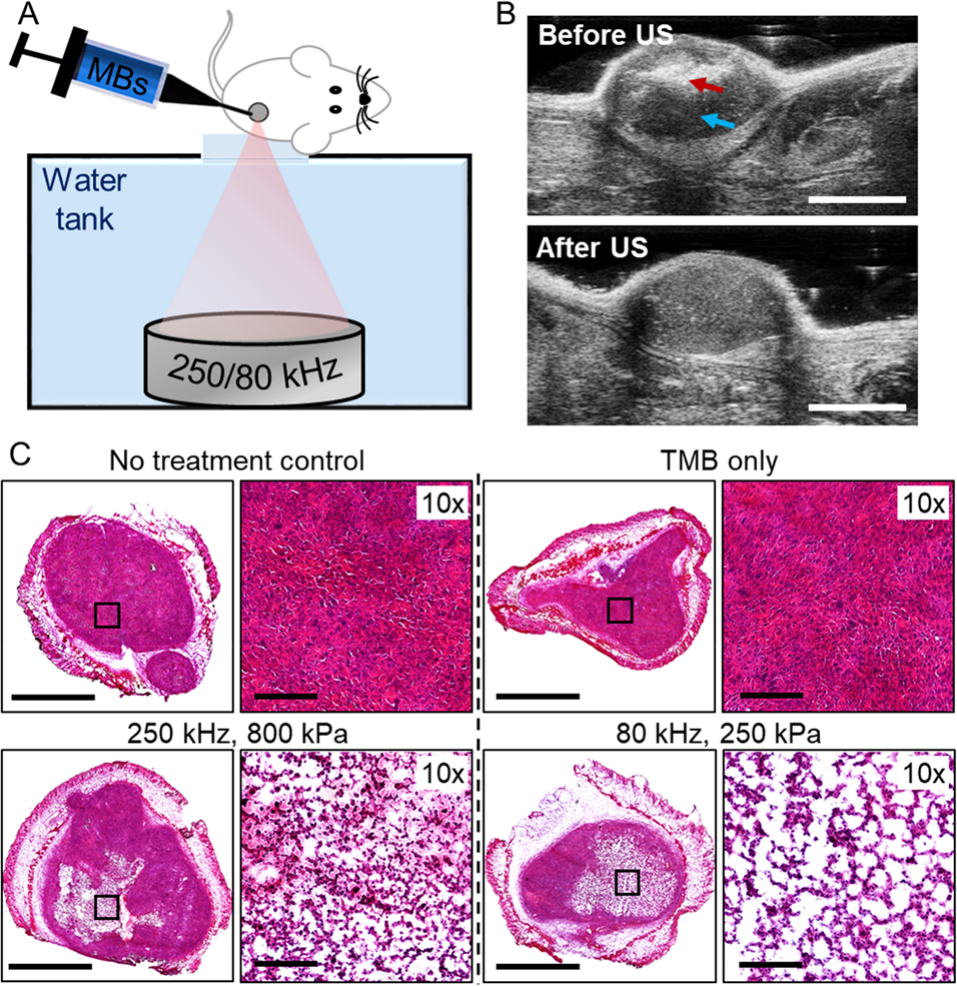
Tumor-targeted microbubbles combined with low frequency ultrasound
